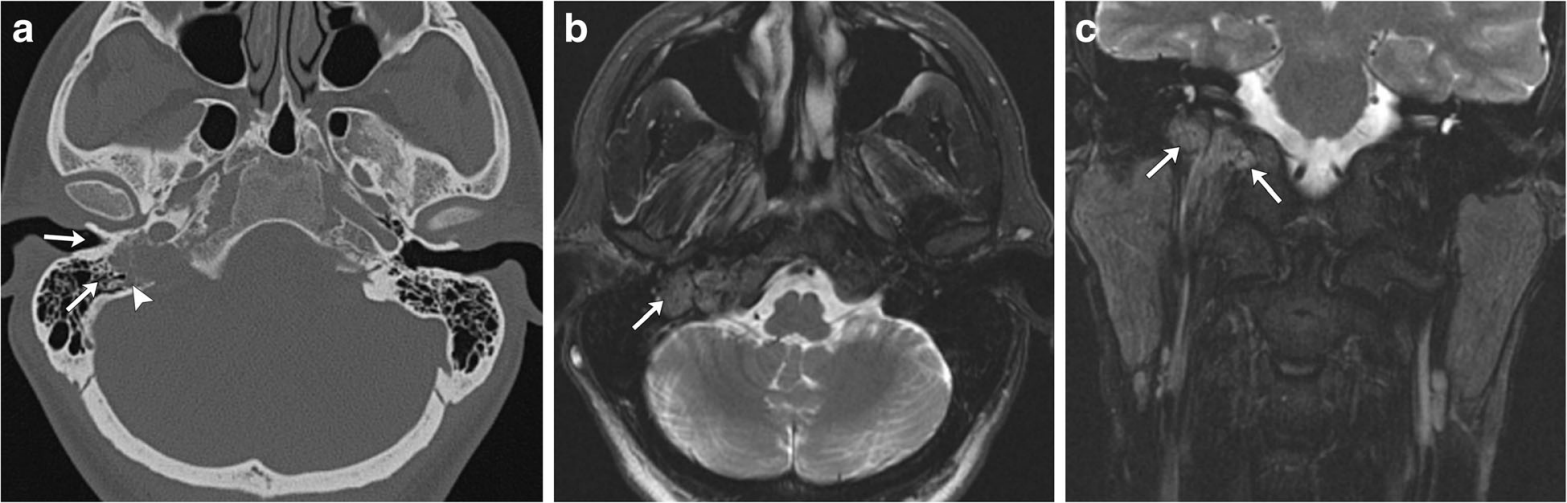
Axial CT (a) demonstrates erosion of the right jugular bulb, with a moth-eaten appearance of the walls of the right jugular foramen (white arrowhead). There is no invasion or extension into the middle ear, as the cochlear promontory remains intact (white arrows). Axial (b) and coronal (c) T2-weighted FSE MR images demonstrate a well-defined, predominantly T2-hyperintense, expansile lesion centered in the right jugular foramen (white arrows). Characteristic “salt and pepper” appearance is present, with punctate regions of hyperintensity representing the “salt,” while small flow voids represent the “pepper.” No extension into the middle ear is seen
